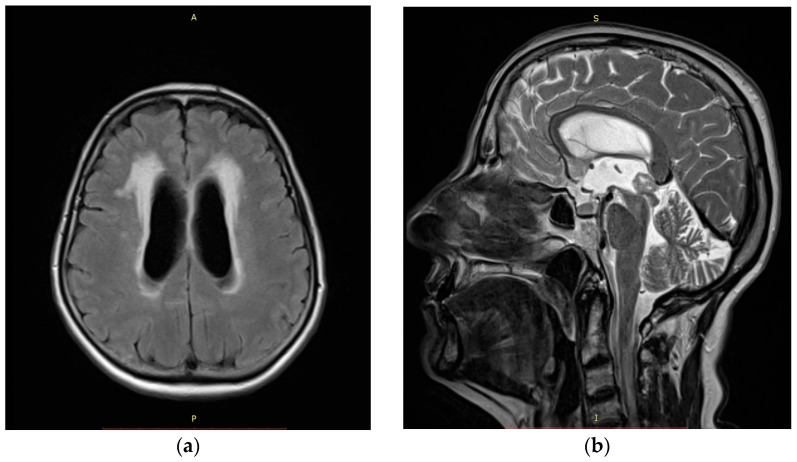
(a) Axial plane T2-weighted-fluid-attenuated inversion recovery (FLAIR) MRI scan of patient with pineocytoma and radiological signs of obstructive hydrocephalus included in our study. (b) Sagittal plane T2 fast spin echo (FSE) MRI scan in the same patient.
